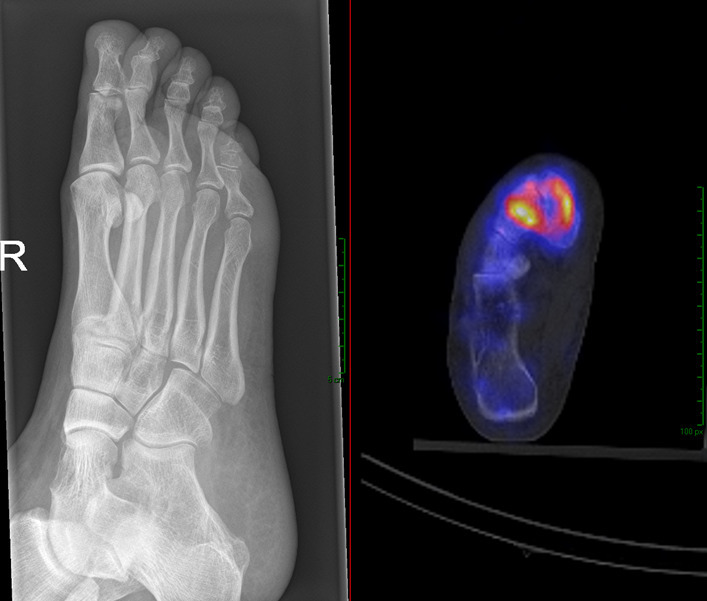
This patient (female of 50 years old) was treated 2 years before presenting for a stress fracture of the second metatarsal. However, her pain was persistent. On examination there was a mild swelling over the midfoot and there was tenderness when applying pressure at the midfoot. The X-rays did not show any abnormalities. The observer scored this case initially as no arthrosis. After assessing the SPECT/CT, the diagnosis was changed in navicular cuneiform OA and tarsometatarsal OA of the first ray. Before access to the SPECT/CT, the observer prescribed painkillers, after the SPECT/CT the plan was an ultrasound guided injection.
